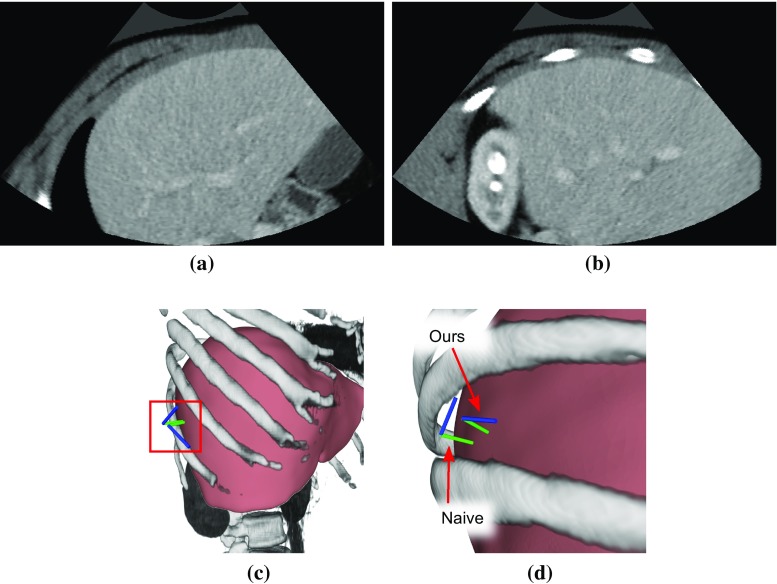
Comparison of synthetic poses for one target point. a Shows the CT slice at the proposed pose, b at the one resulting from naive planning. c, d Set the poses in relation to the surrounding anatomy
